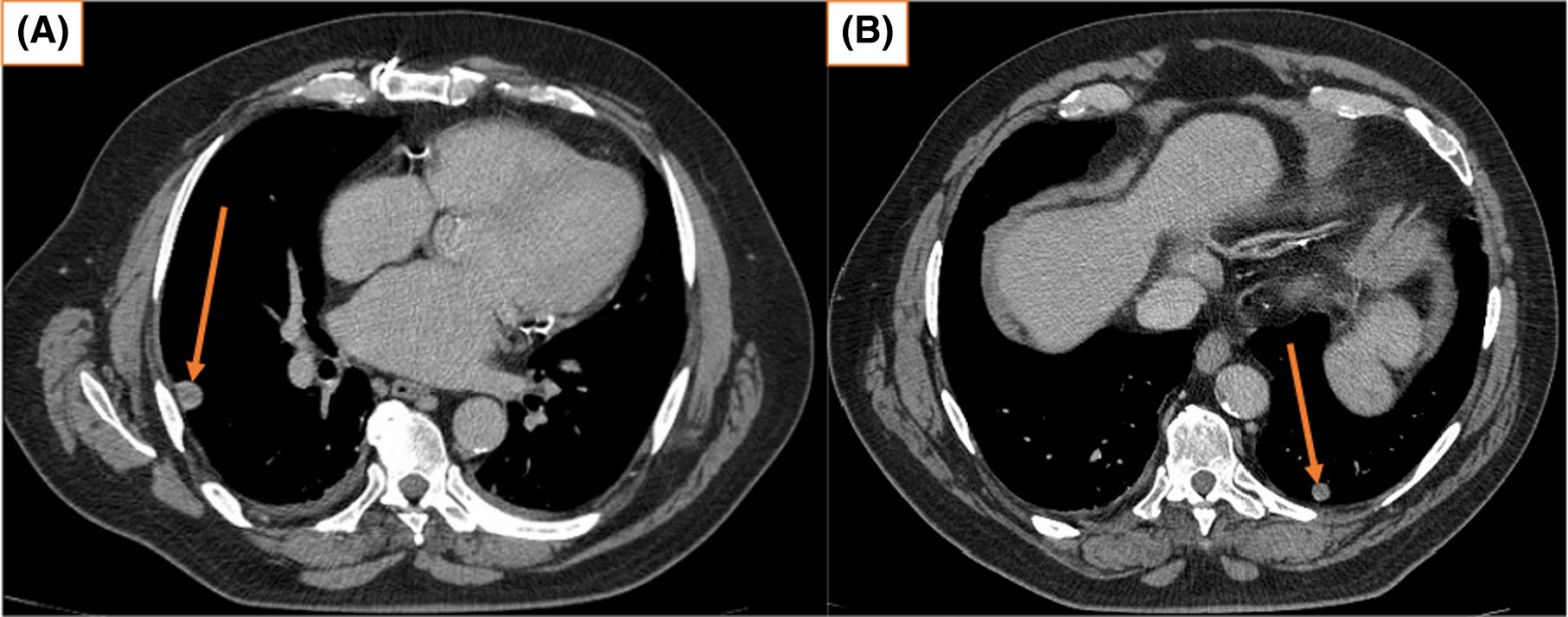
(A) May 2022, chest CT showing an indeterminate 13 mm pleural nodule in the right lower lobe, suggestive of right pleural metastasis (pre‐orchiectomy). (B) May 2022 chest CT showing a 9 mm pulmonary nodule in the left lower lobe, suggestive of left lung metastasis (pre‐orchiectomy).
